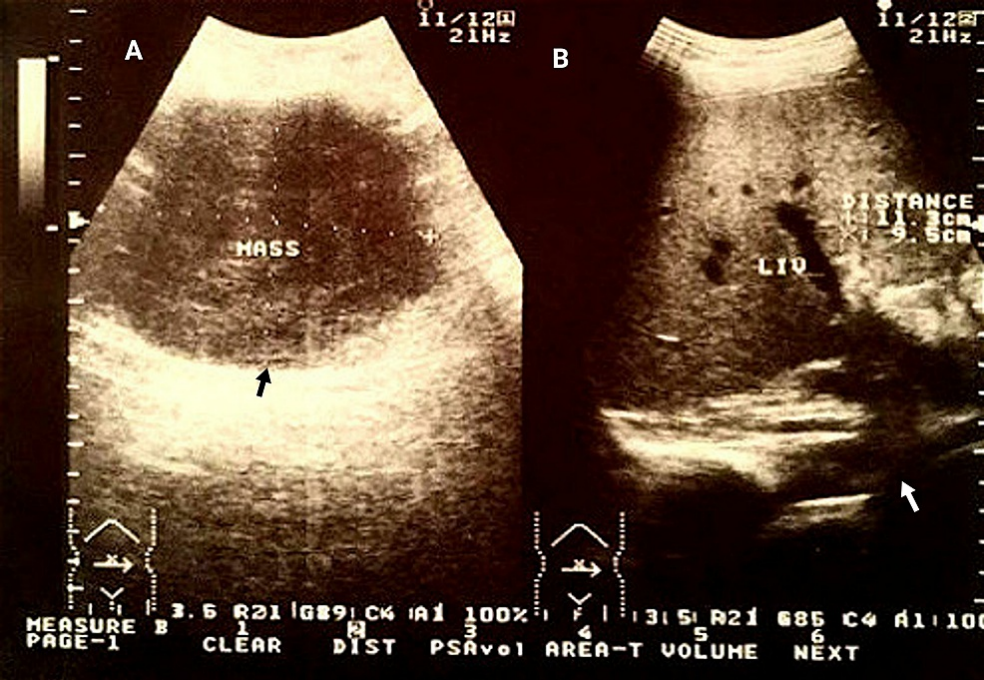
Ultrasound of the abdomen A: Black arrow showing a large mass in the abdomen;  B: White arrow showing accumulation of ascitic fluid in the abdominal cavity.
